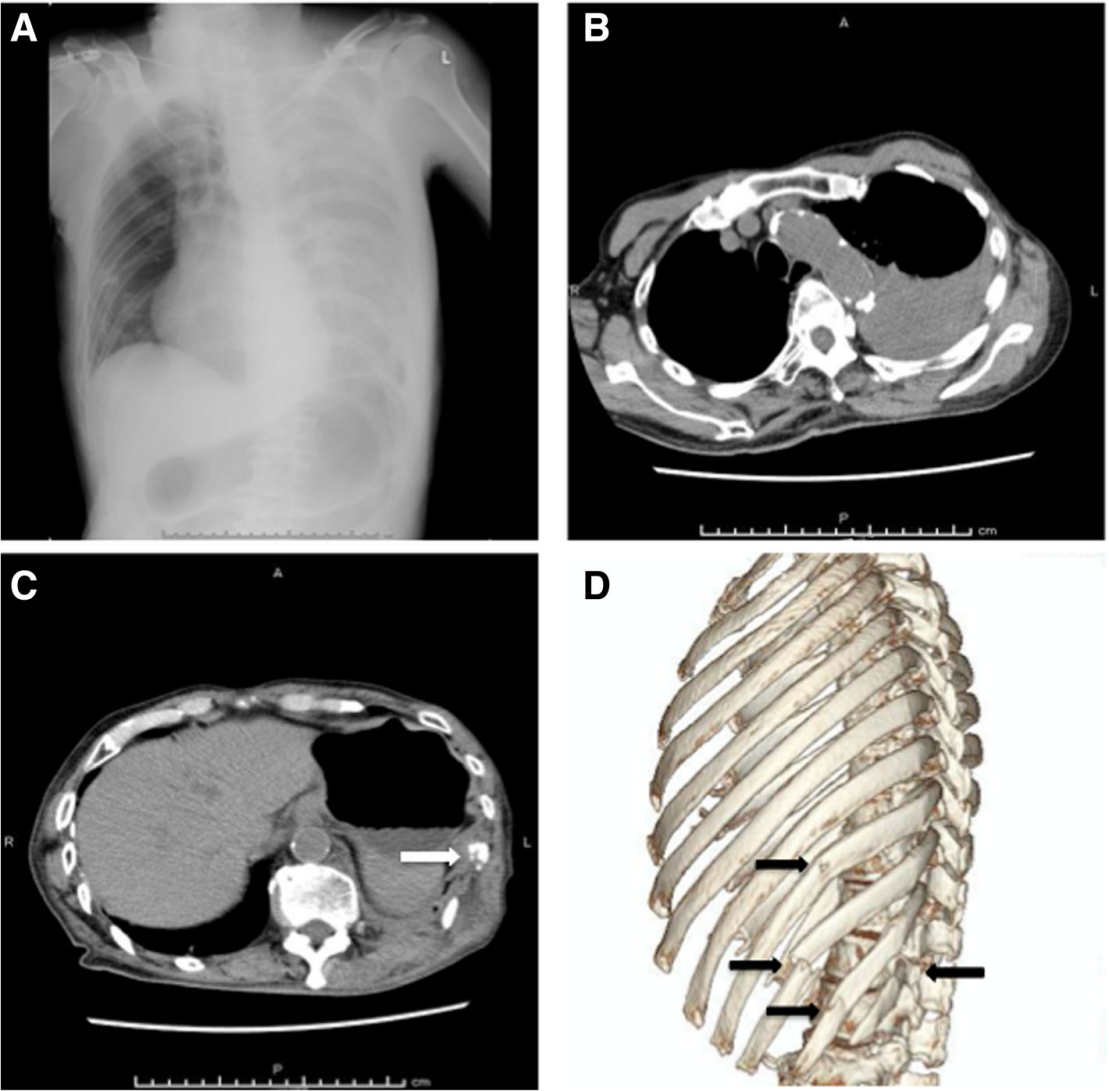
Chest radiography and computed tomography imaging findings of the patient. Chest radiography on arrival at emergency room shows left hemothorax (a). First computed tomography images show hemothorax (b) and fracture of tenth rib (c). Multiple fractures of 9th to 12th ribs (*arrow*) are revealed by (d)
